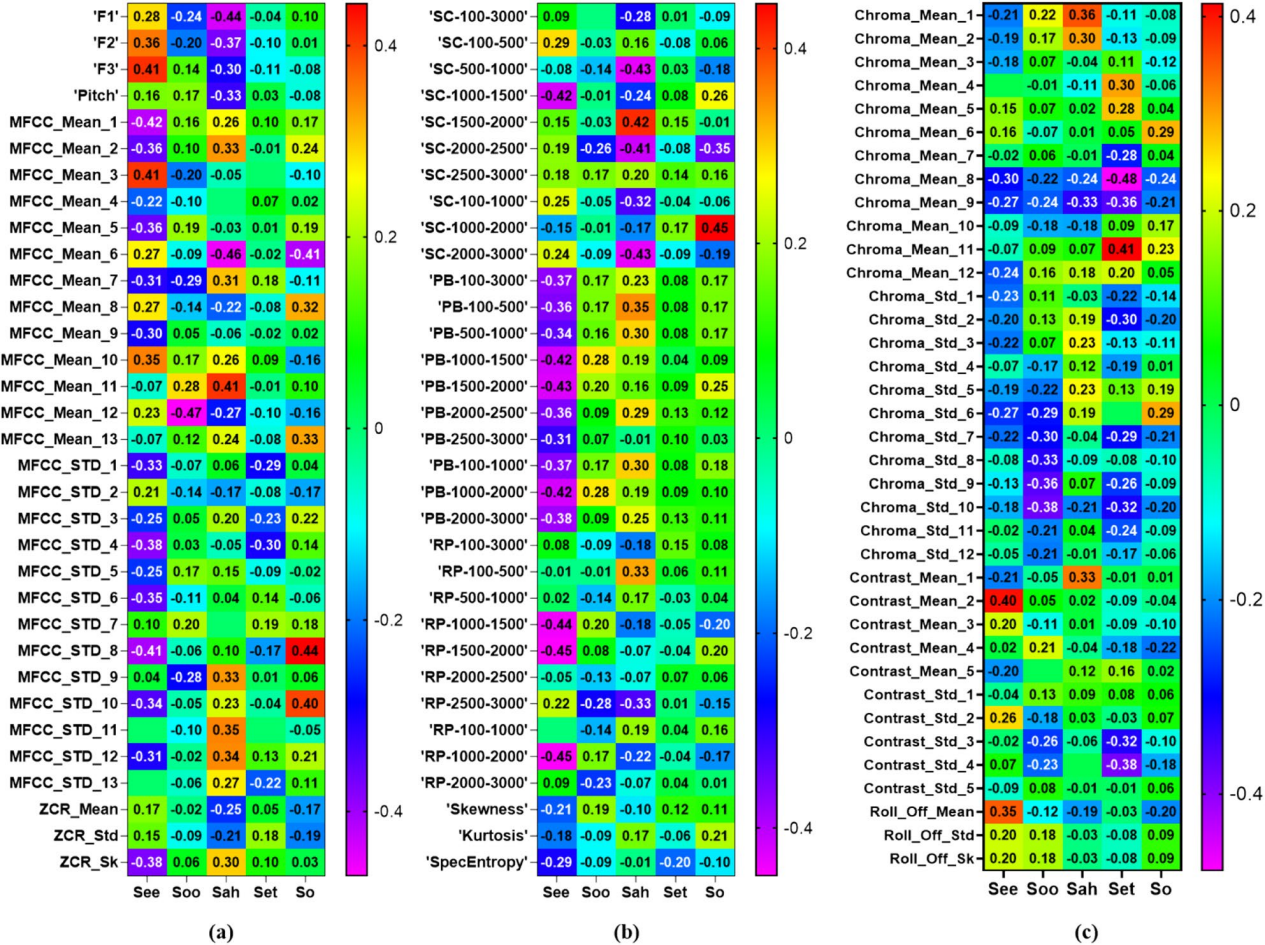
Heatmap of the correlation coefficients between the ultrasonography measured parasagittal anterior posterior (PAP) diameter  and acoustic features of vowel sounds: (a) pitch, formants (F1, F2, F3), mel frequency cepstrum coefficient (MFCC)–mean and standard deviation, and Zero crossing rate (ZCR), (b) spectral centroid (SC), band power (PB), relative power (RP) in 10 frequency bands, skewness, kurtosis, and entropy (SpecEntropy), (c) chroma, contrast, and roll-off frequency. (Created by GraphPad Prism: https://www.graphpad.com/).
